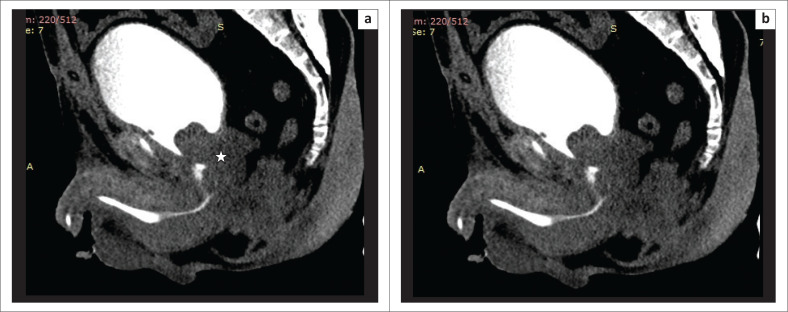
Multidetector computed tomographic urethrography: Sagittal multiplanar reformatted image showing prostatomegaly (star), with the median lobe seen bulging into the bladder base and compressing the posterior urethra, creating a false impression of a posterior urethral stricture.
